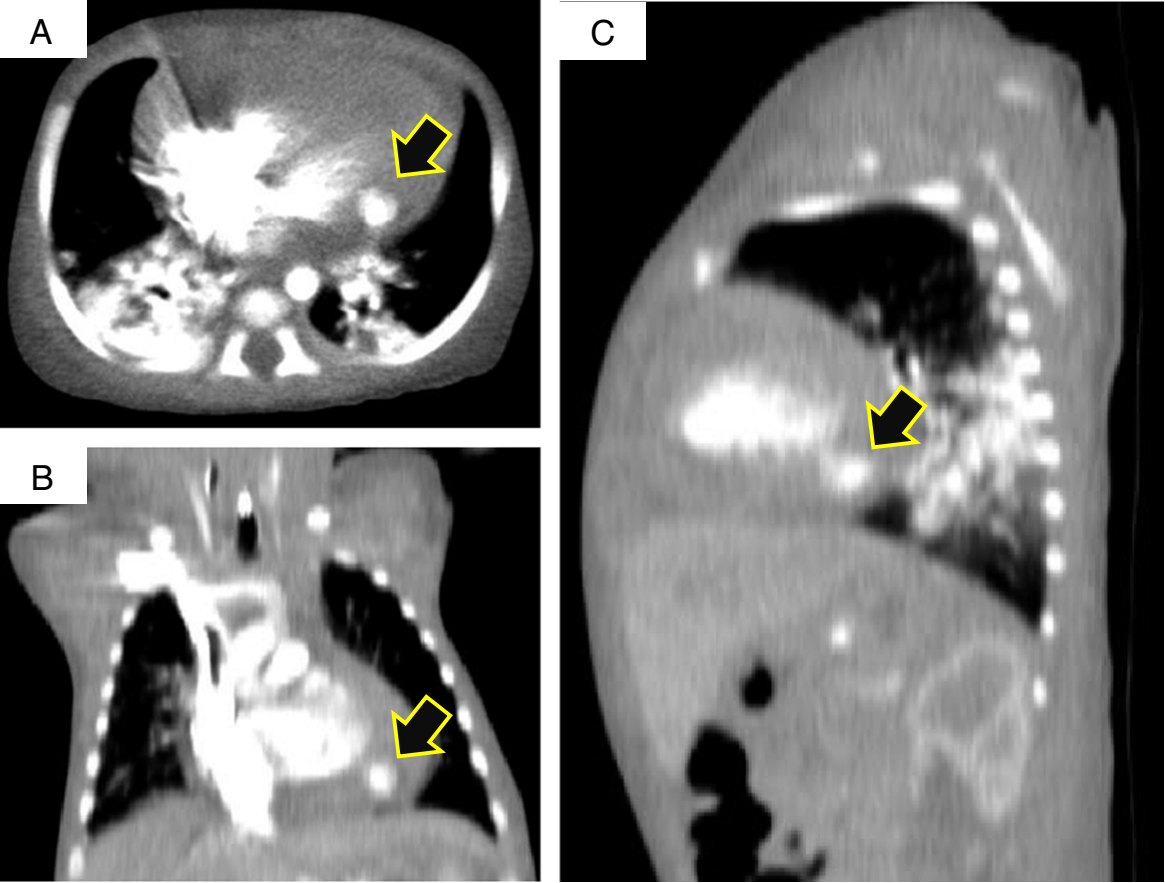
Enhanced computed tomography scan showing a contrast-filled left ventricular outpouching (arrows); with in an axial view (A), in a coronal view (B), and in an oblique view (C).
